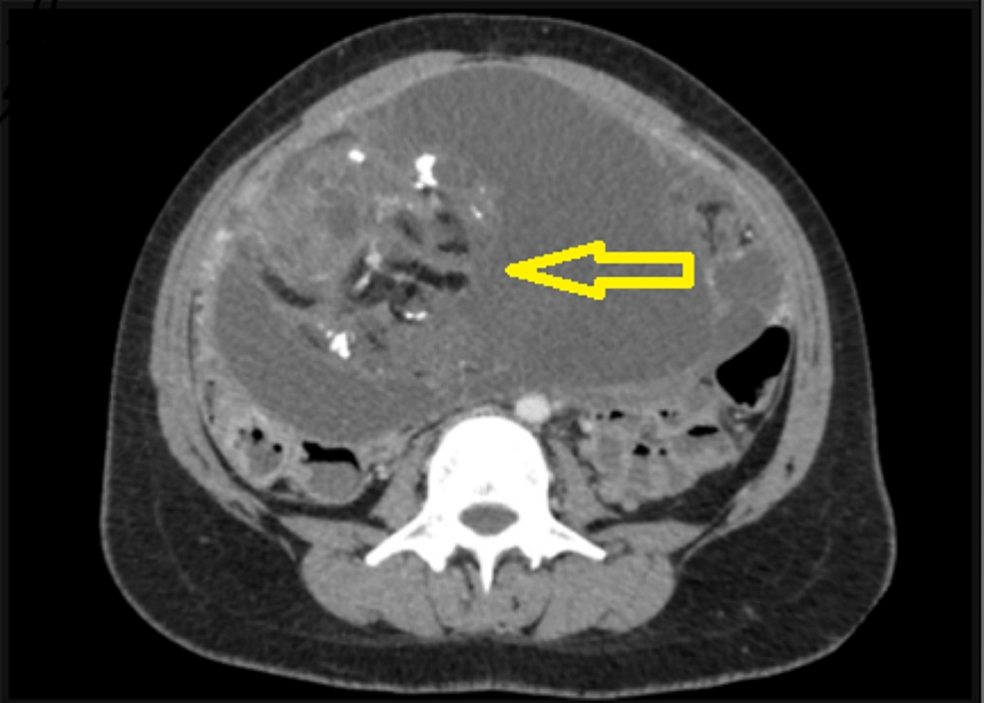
Contrast-enhanced computed tomography of the abdomen and pelvis showed a large well-defined heterogeneously enhancing lesion of size 85 x 75 x 90 mm in the lower abdominopelvic region. The lesion shows fat density, solid components, and calcific foci within it.
